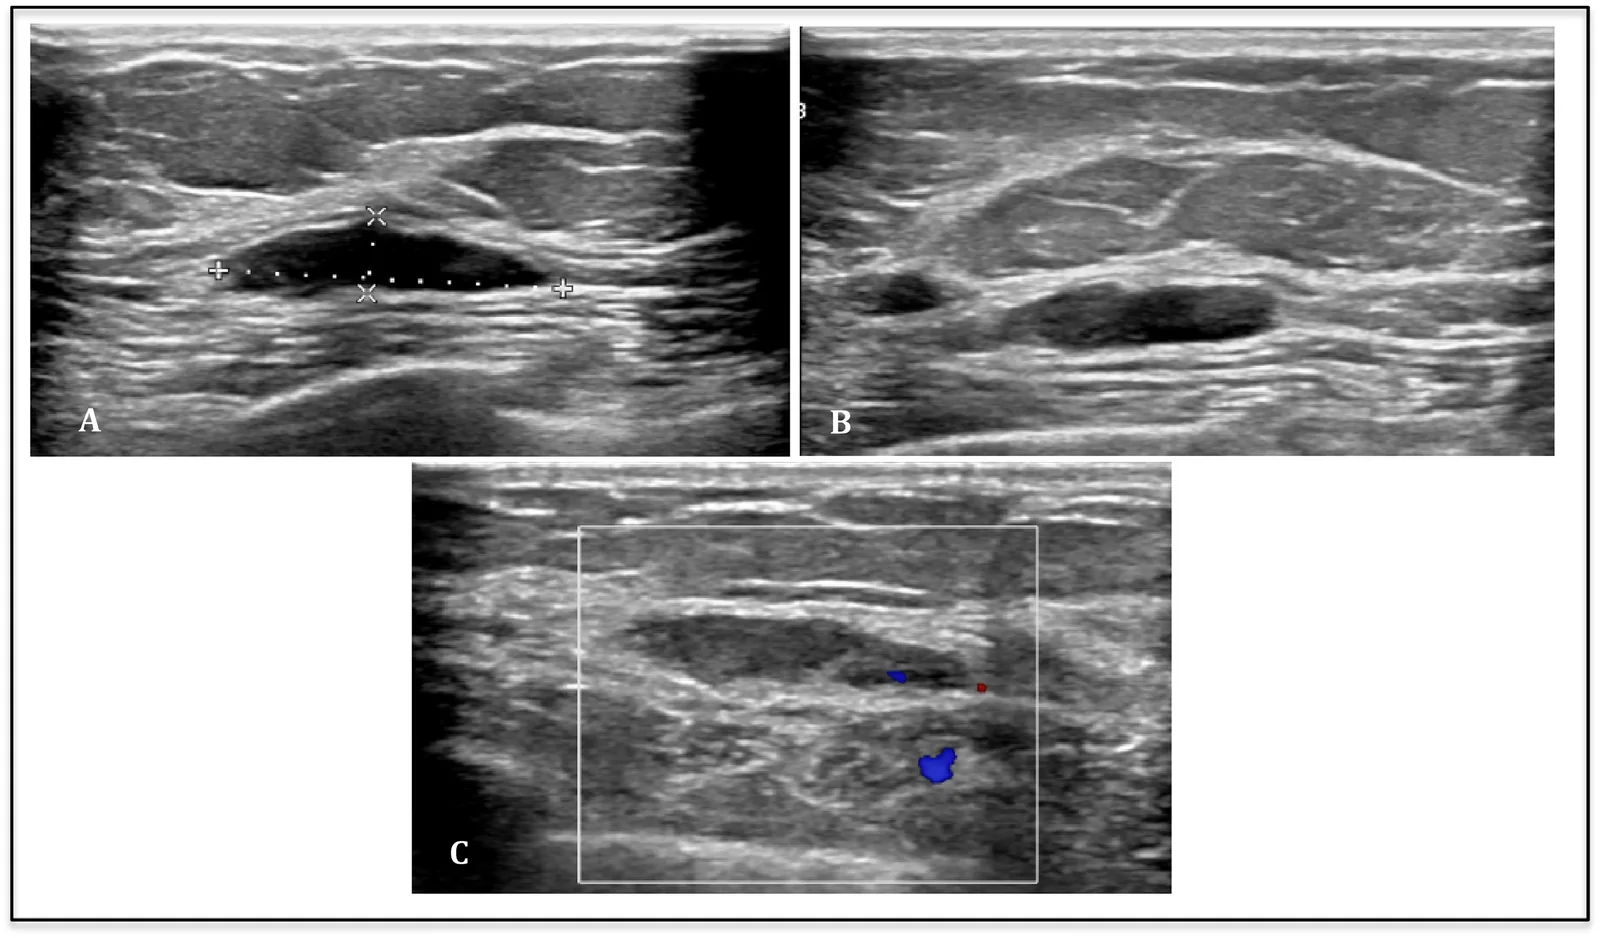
Breast ultrasound demonstrated multiple bilateral oblong masses, well circumscribed with smooth regular margins, exhibiting minimal internal vascularity on color Doppler imaging. (A) upper inner quadrant of the left breast; (B) lower inner quadrant of the right breast; (C) color Doppler.
